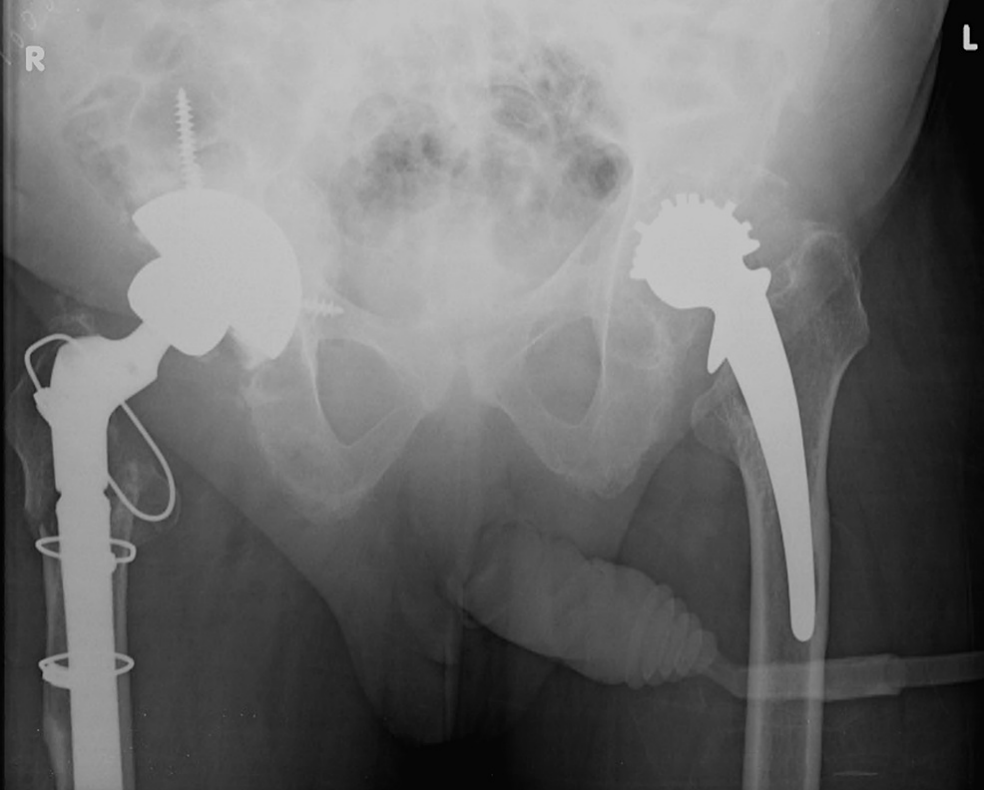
Postoperative pelvis X-rays after right revision total hip arthroplasty with an uncemented acetabular shell and a long uncemented diaphyseal engaging femoral stem
